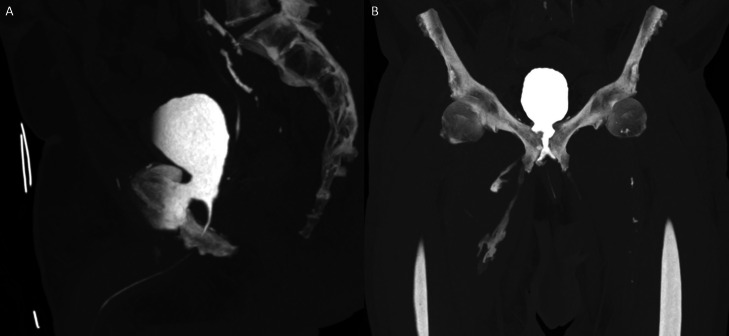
Delayed-phase CT scan performed 90 minutes later. (A) Sagittal slice demonstrating a direct fistulous tract between the anterior-inferior bladder wall and the pubic symphysis. (B) Coronal slice showing fistulous tracts extending into the bilateral adductor compartments.
